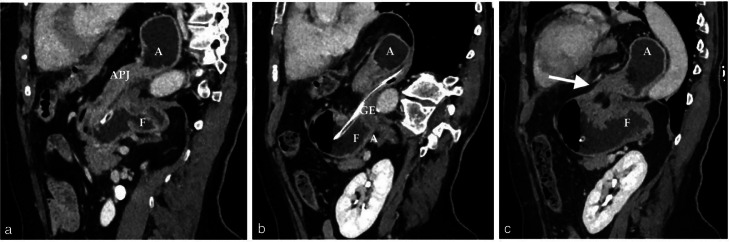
(A-C) Sagittal MRP CT reconstruction. Gastric fundus (F) is located below the left hemidiaphragm, the antrum (A) is displaced cranially into the thorax. The gastroesophageal junction with nasogastric tube (GE) lies posteriorly. The antropyloric junction (APJ) is displaced anteriorly. Gastric stenosis is clearly visible at the hernial neck (white arrow).
